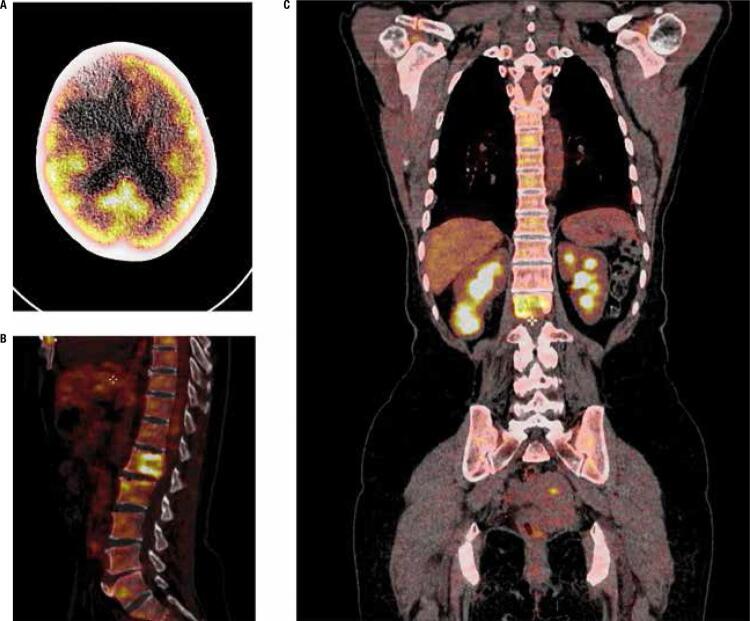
18F-FDG PET/CT images show increased metabolic activity in 62-y-woman: A. Expansive lesion on frontal lobe of the brain; B. Lytic lesion on L2 vertebral body; C. Right lower renal mass. (Table 3, patient 8).
